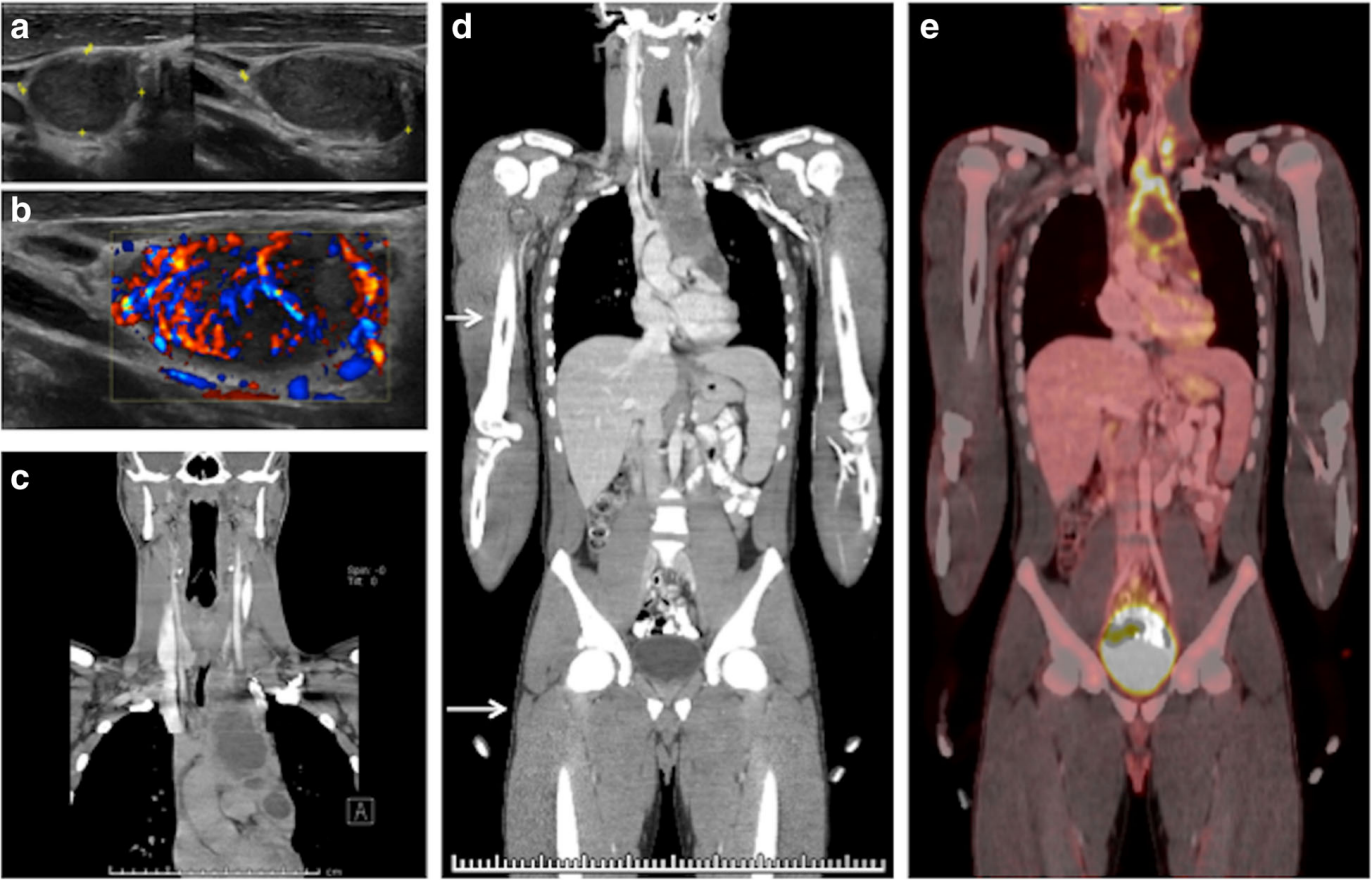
Multi-series PET/CT with Dx Abdomen/Pelvis CT. Eighteen years old patient with cervical adenopathy was evaluated initially by US (a, b). Subsequent evaluation included a diagnostic CT of the neck and chest (c). Biopsy revealed Hodgkin lymphoma. Completion staging involved a diagnostic CT of the abdomen and pelvis, which was incorporated into the PET/CT acquisition (d, e), to avoid repeat diagnostic CT imaging of the neck/chest and double scanning of the abdomen and pelvis. Arrows (d) show the junction between low dose AC CT and diagnostic portions of the exam
